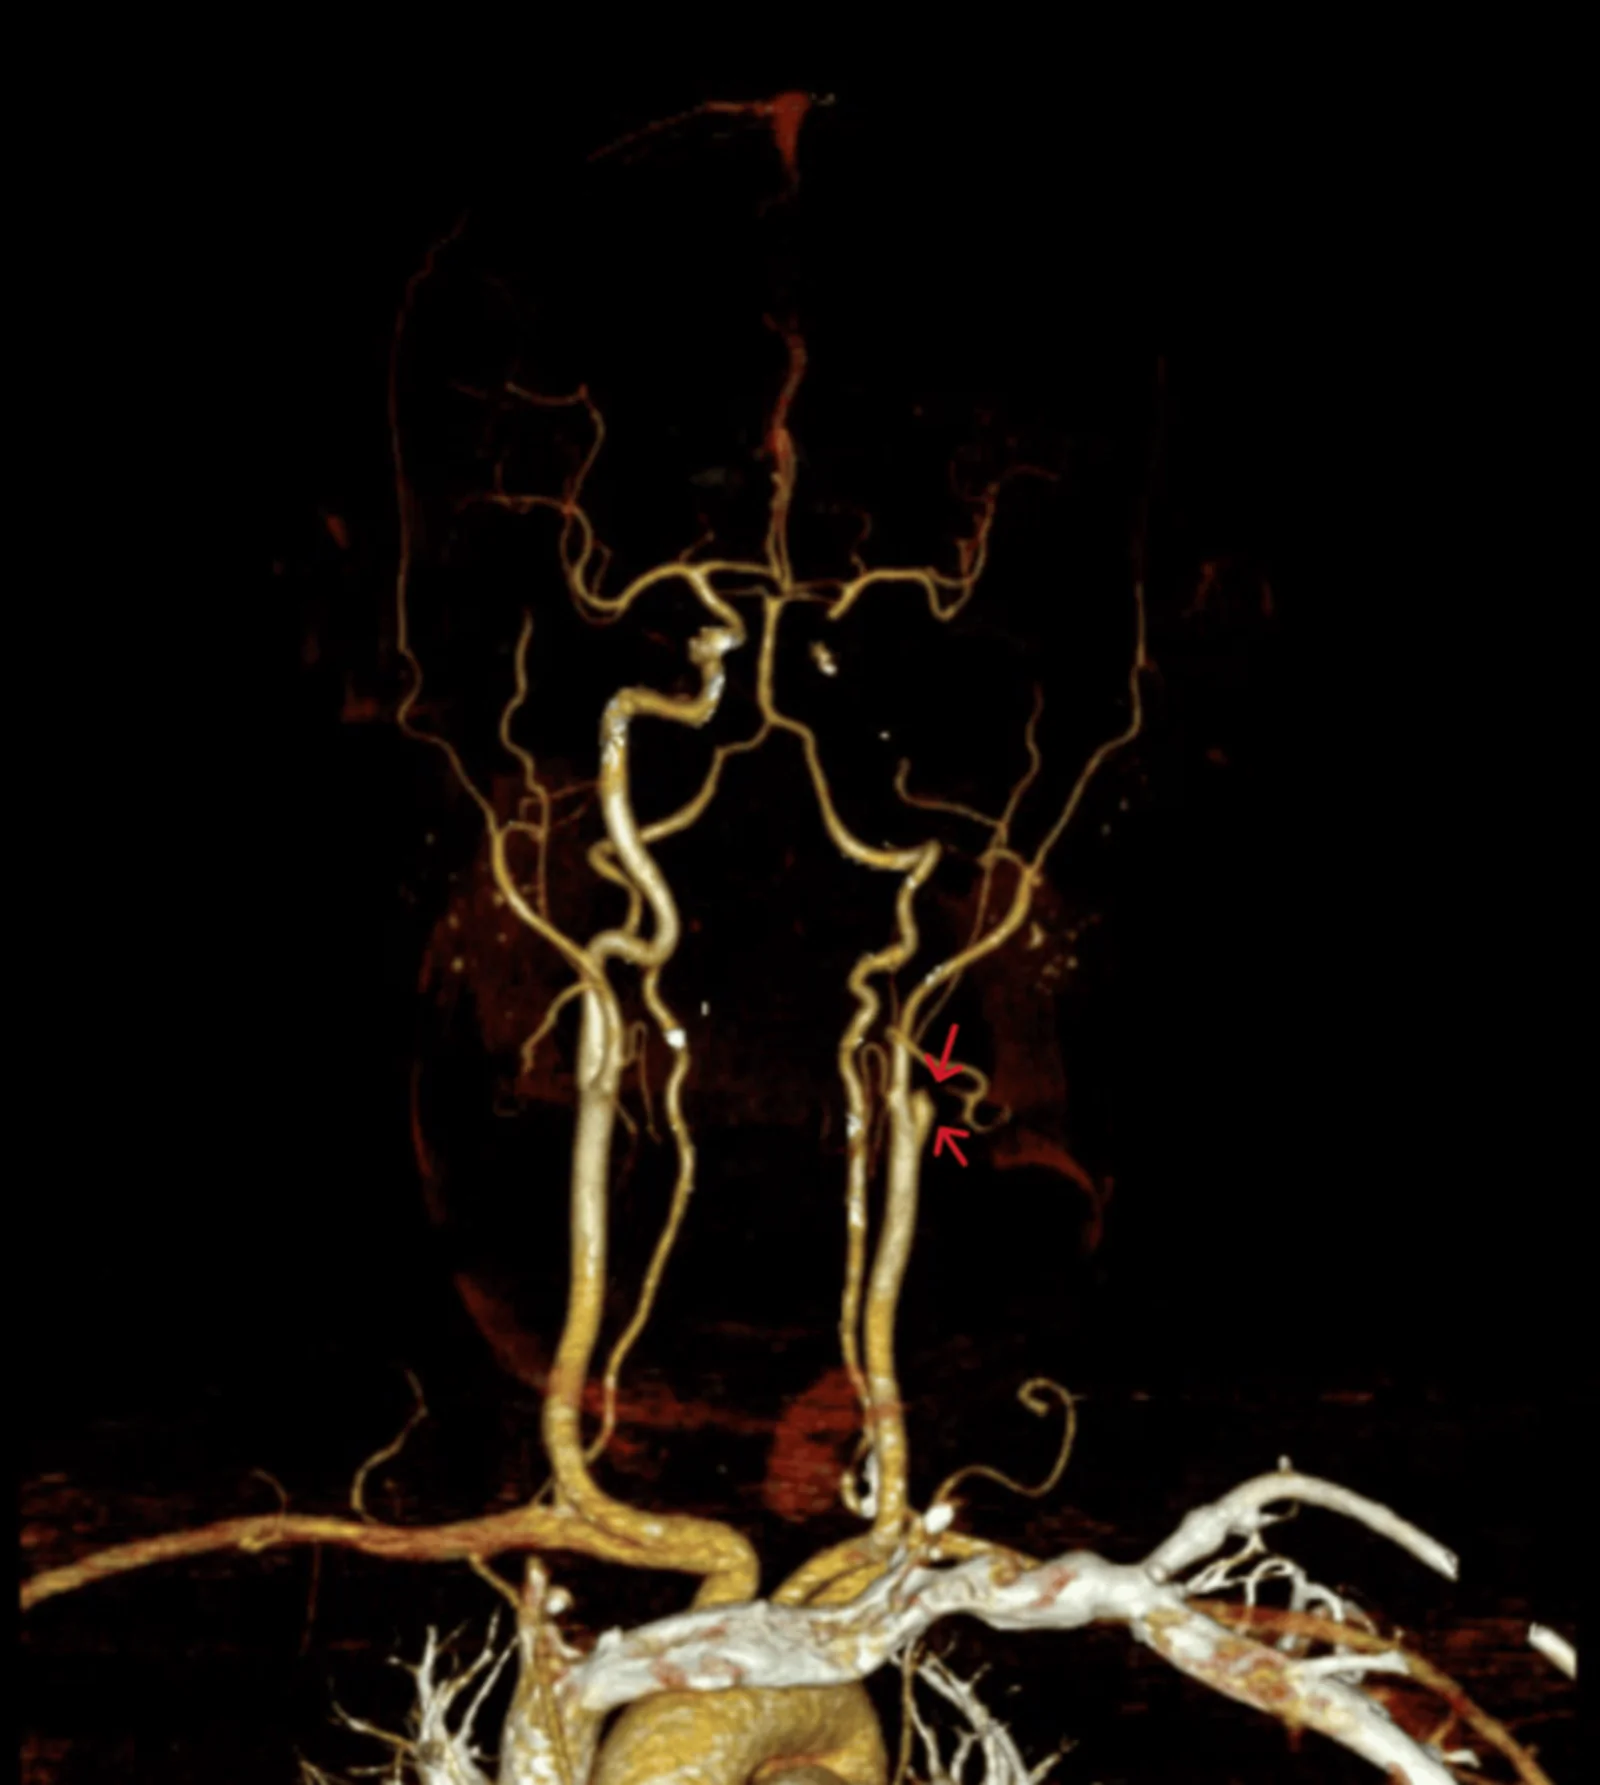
CT angiography (CTA) of the head and neck demonstrating complete occlusion of the left internal carotid artery from just distal to its origin, involving the cervical, petrous, cavernous, and terminal segments (as indicated by red arrows).
